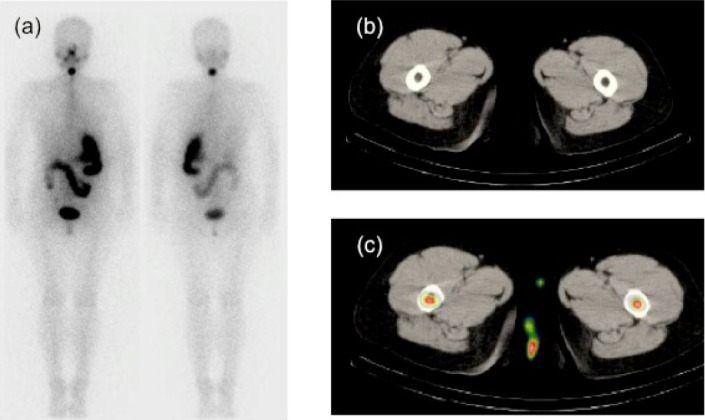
A -41year-old female patient with type B uptake in the initial RAI therapy (3.7 GBq) (a). Axial CT (b) and fused SPECT/CT (c) images show an uptake, localized in the bilateral bone marrow of the femur.
